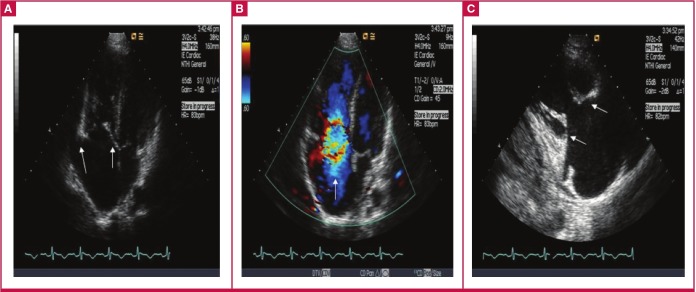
Patient 2. (A) Apical four-chamber view: note the thickened, immobile and retracted tricuspid leaflets and minimally thickened mitral valve leaflets (arrows), and the dilated right atrium and ventricle. (B) Torrential tricuspid regurgitation (note the arrow). (C) Marked failure of coaptation (indicated by arrows) of the tricuspid valve leaflets.
